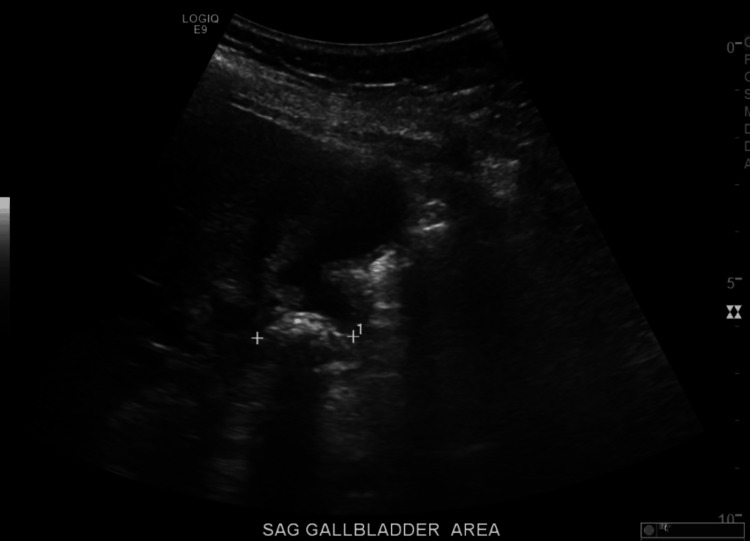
Ultrasound abdomen showing gallbladder stone with dilatation of the common bile duct.
